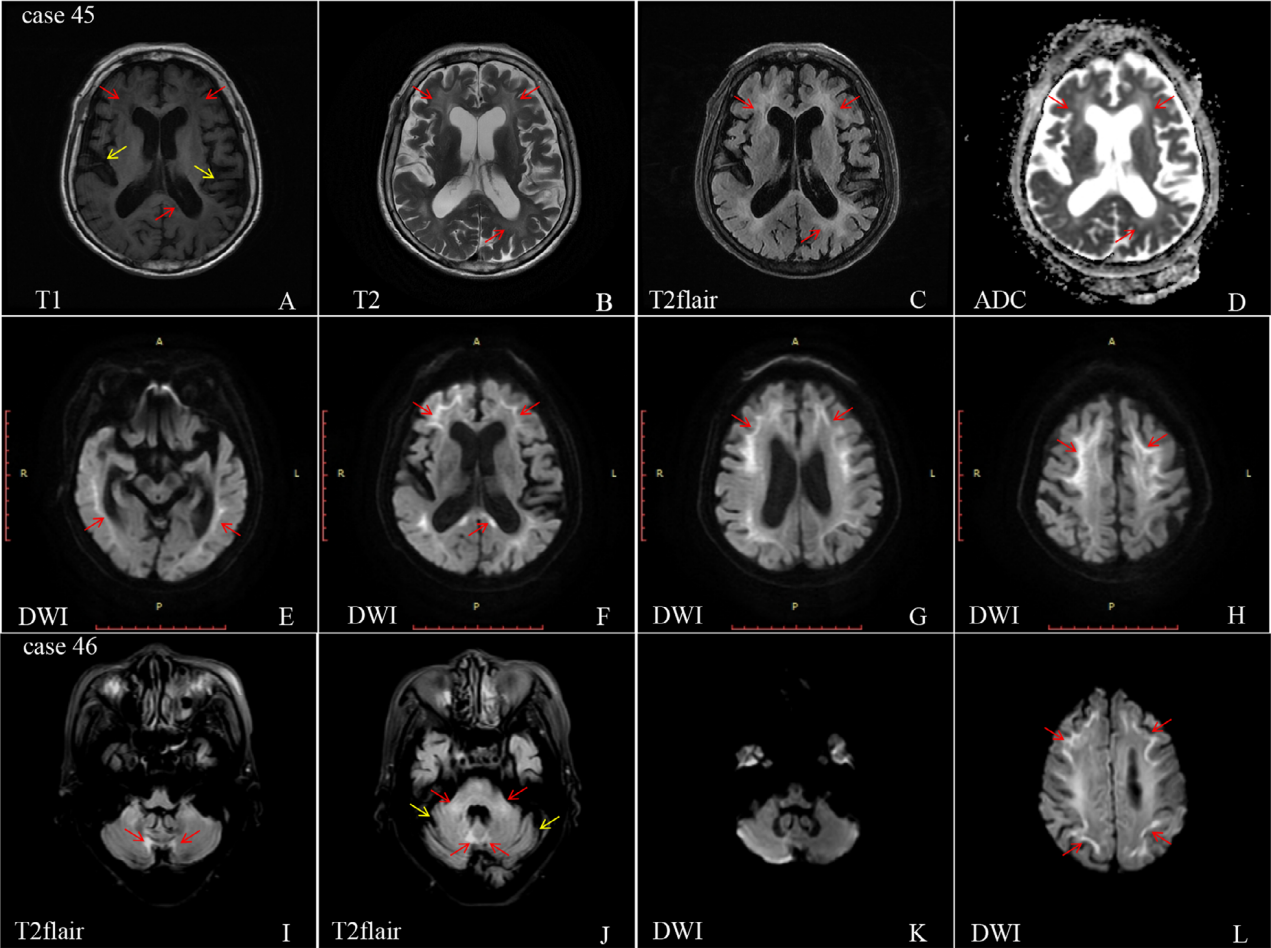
Typical features of MRI in patients with NIID. A representative case (case 45, A–H) shows the typical imaging manifestations showed T1 low signals (red arrows) and high signals in T2, FLAIR, and ADC axial images involving the corticomedullary junction of frontal lobe, parietal lobe, occipital lobe, temporal lobe, and corpus callosum (A–D). DWI shows ribbon signs imaging manifestations involving the corticomedullary junction, accompanied by cerebral atrophy, mainly in the temporal lobe and hippocampus (E–H). Another representative case (case 46, I–L) showed bilateral high signal intensity in the medial part of the cerebellar hemisphere immediately beside the vermis (the paravermal area, I) and in the middle cerebellar peduncle (red arrows, J) as well as the atrophy of the cerebellum (yellow arrows, J). DWI axial images showed no obvious abnormality in the cerebellum (K); however, obvious high signal intensity was found along the corticomedullary junction (L).
